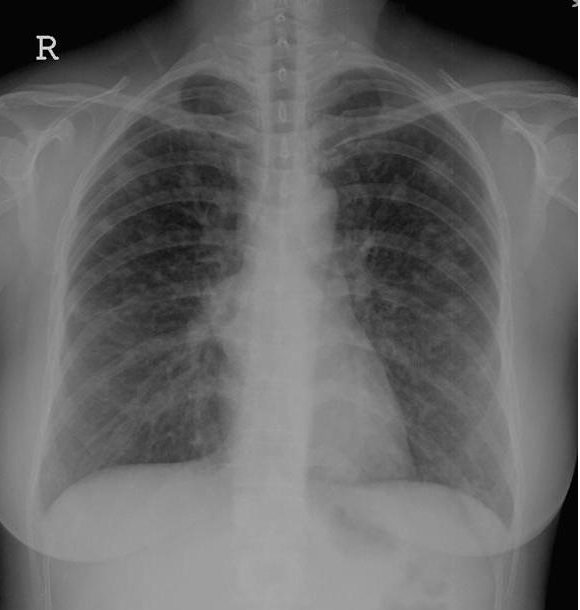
The chest X-ray revealed multiple pulmonary nodular lesions.
